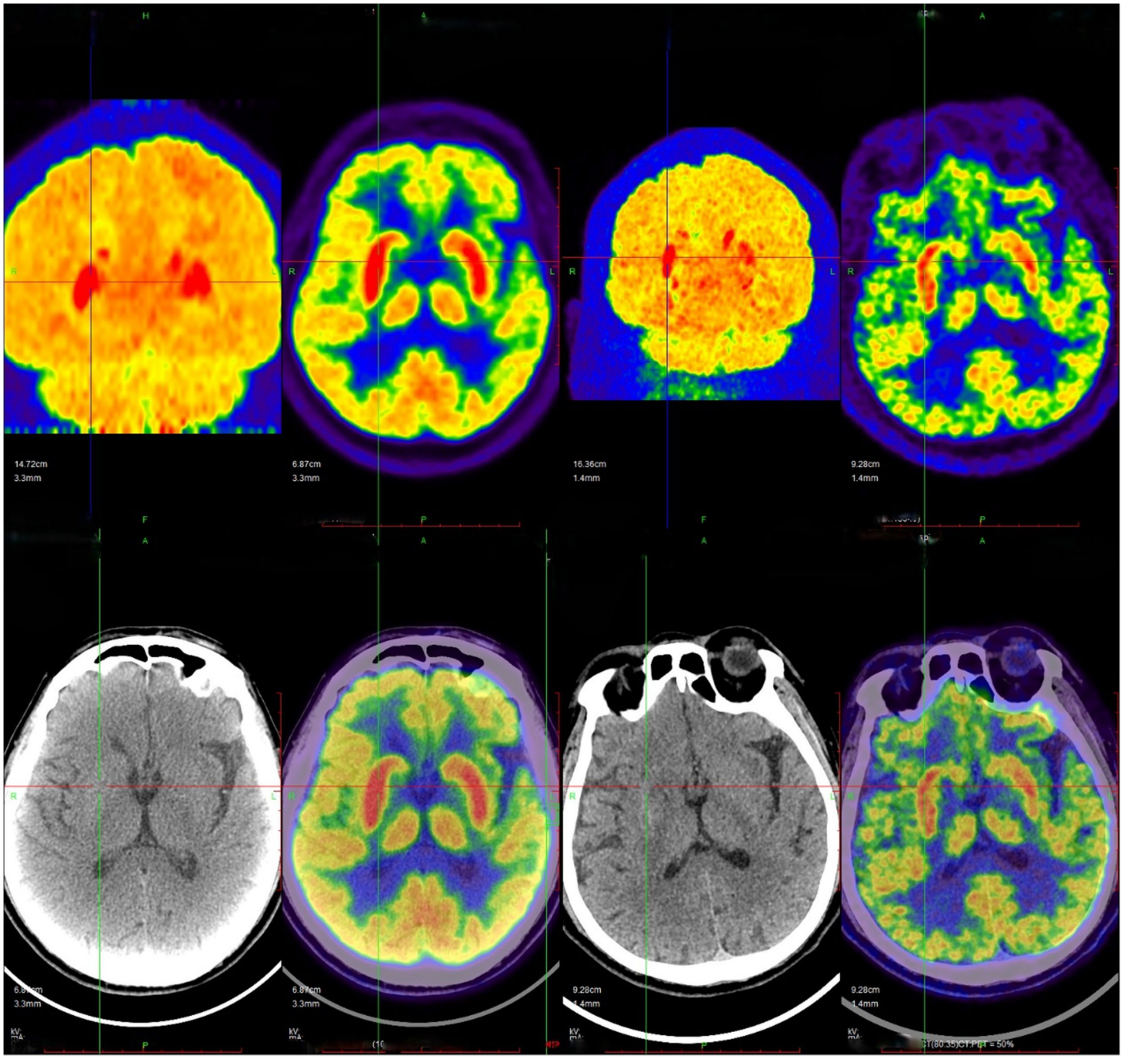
18F-FDG PET/CT images of the patient with anti-LGI1 encephalitis. The images demonstrate elevated metabolism in the bilateral basal ganglia, with a more pronounced increase on the right side. Additionally, there is a mildly increased metabolism in the bilateral hippocampi, with a relatively more notable elevation on the right side.
